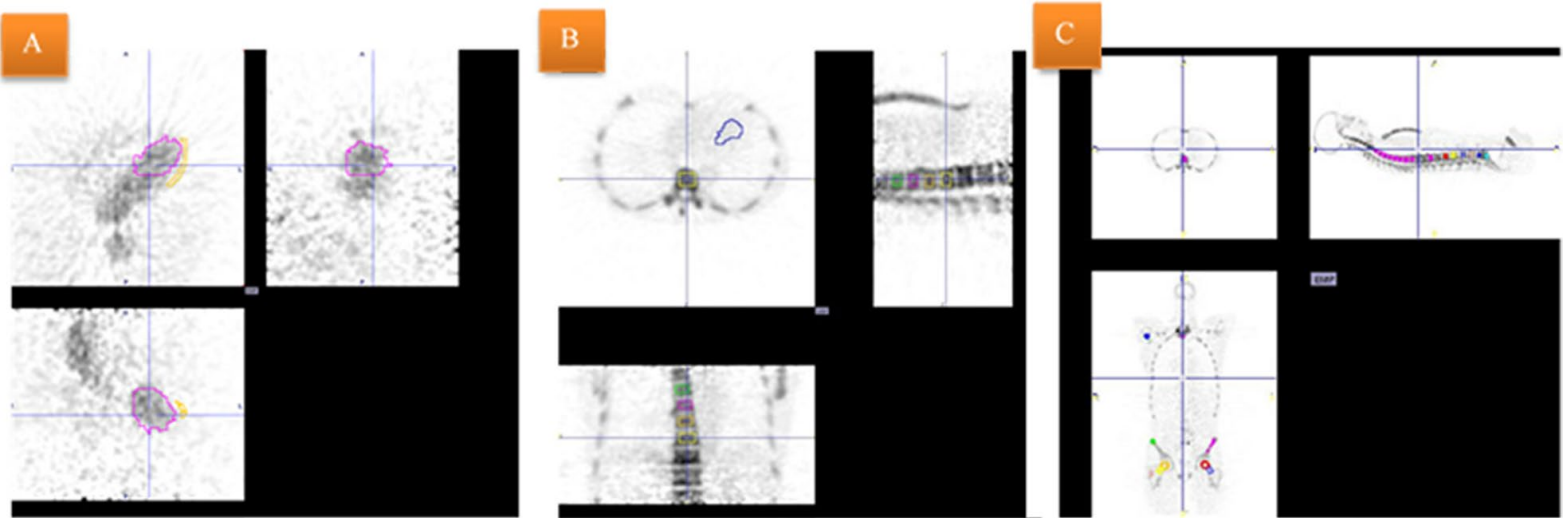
A A VOI placed in LV and a myocardial VOI for β-correction. B FOV from a dynamic ^18^F-NaF PET/CT showing VOIs to obtain bone TACs in vertebrae Th7–10 and a VOI in the left ventricle of the heart (LV) to obtain the image-derived input function. C Whole-body ^18^F-NaF PET/CT with the possibility to make bone VOIs in the entire skeleton
